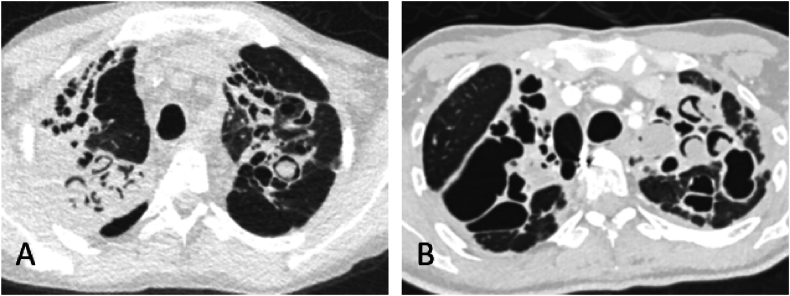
Computed Tomography images of case 1 (A) and case 2 pre-transplantation (B). In both images, severe bronchiectasis with rounded masses inside cavities with the air crescent sign can be seen, typical of fungal infection.
